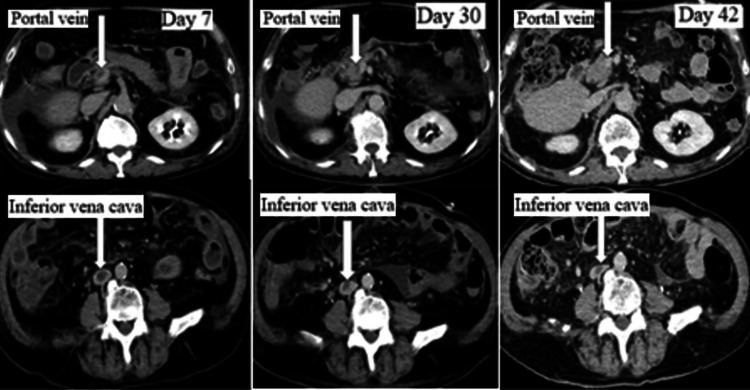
The contrast-enhanced CT images on day 7, day 30, and day 42. Thrombi are observed at the tips of the arrows. CT, computed tomography
